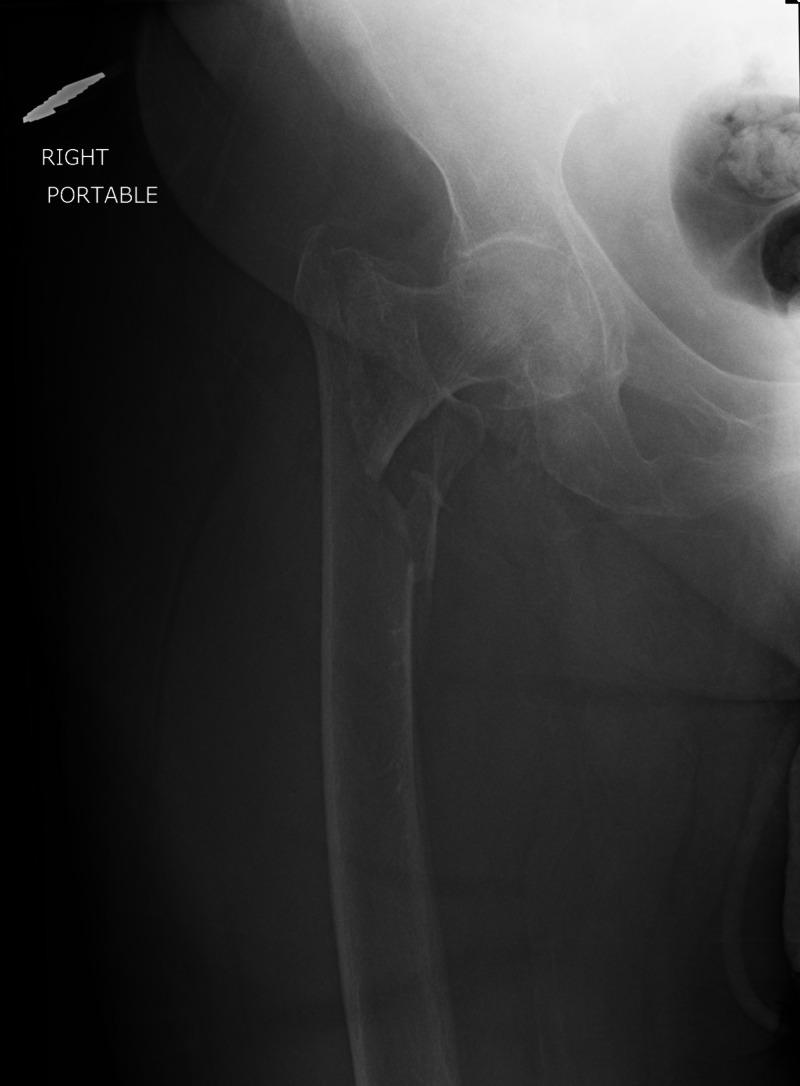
Preoperative Radiograph An anteroposterior radiograph of the right hip demonstrating a displaced intertrochanteric femur fracture with subtrochanteric extension in a patient with a BMI of 31 kg/m^2^
